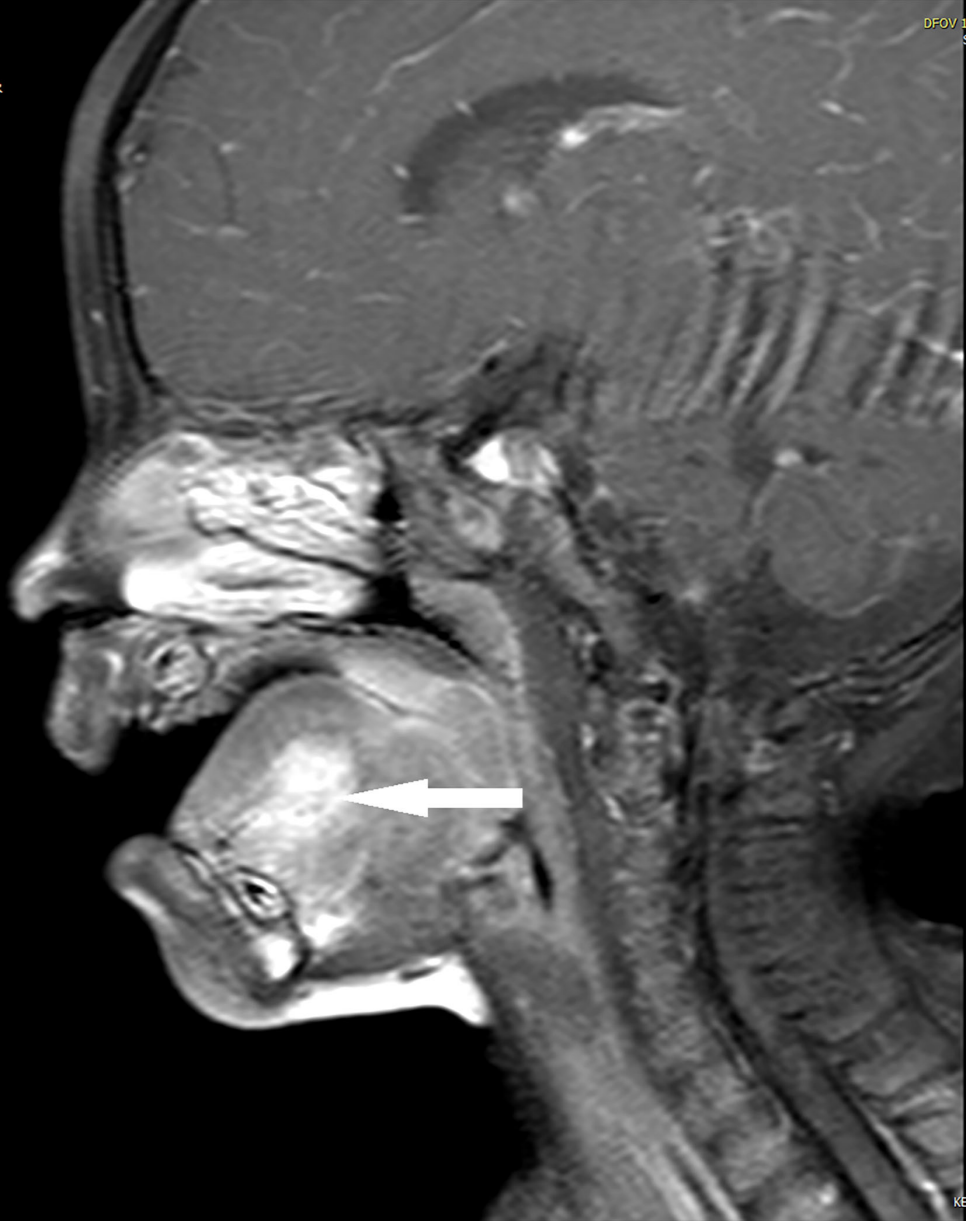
Case 2, tumor in magnetic resonance imaging at relapse (sagittal).
